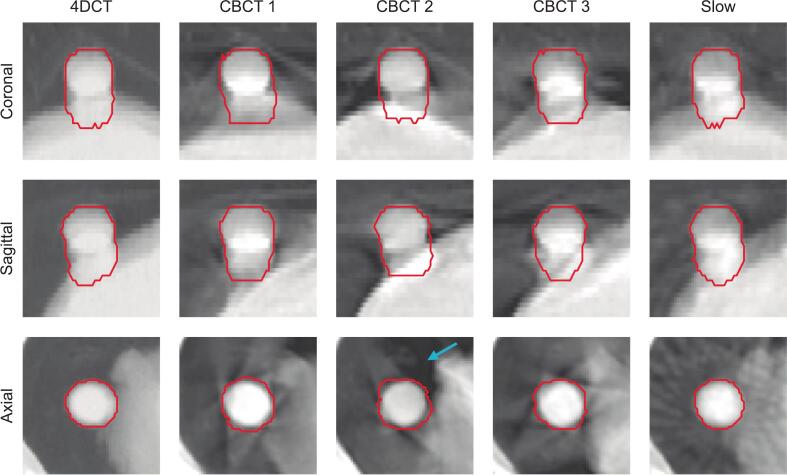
Images and manual contours on the average 4DCT reconstruction, three randomly acquired 6-second HS-CBCT scans (CBCT1-3), as well as the 60-second HS-CBCT scan (Slow), acquired in Trace 1 (cos^6^). The images and contours are displayed in the axial, coronal and sagittal view through the center-of-mass of the contoured tumor. The cyan arrow indicates areas with CT numbers as low as −1000 HU. (For interpretation of the references to colour in this figure legend, the reader is referred to the web version of this article.)
